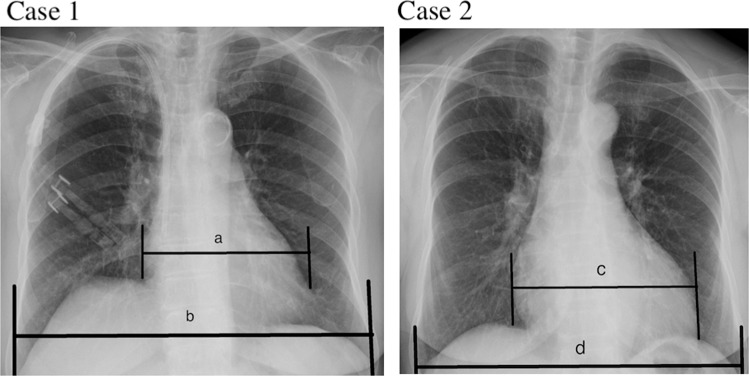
Examples of normal cardiothoracic ratio (CTR) (CTR less than 50%) and abnormal CTR (CTR over 50%) chest X-ray images. (In case 1, the CTR is a/b = 46%; in case 2, the CTR is c/d = 58%).
